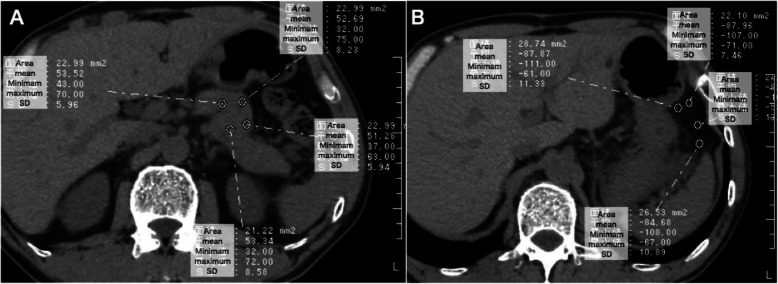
Representative images for the measurements of CT values of the pancreatic parenchyma (a) and visceral fat (b). a Pancreatic CT values in the future remnant pancreatic body-tail are measured in the four different ROIs area of 15 to 30 mm2 on a magnified CT image. b Visceral fat CT values at lateral to the stomach are measured in the four different ROIs area of 15 to 30 mm2. ROI: region of interest, SD: standard deviation
